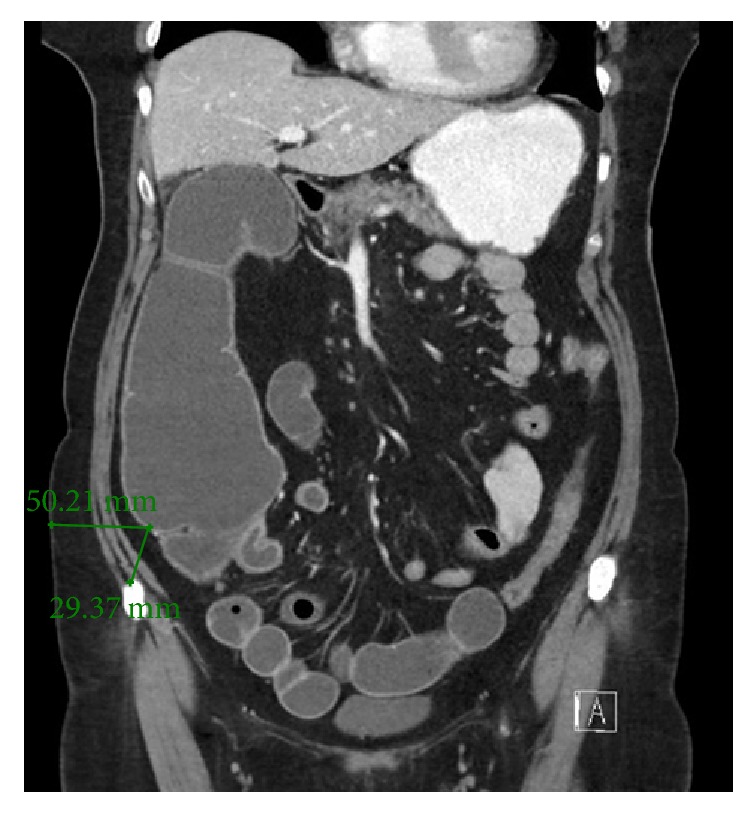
Coronal CT showing caecum measured from the anterior superior iliac spine and the lateral abdominal wall.
